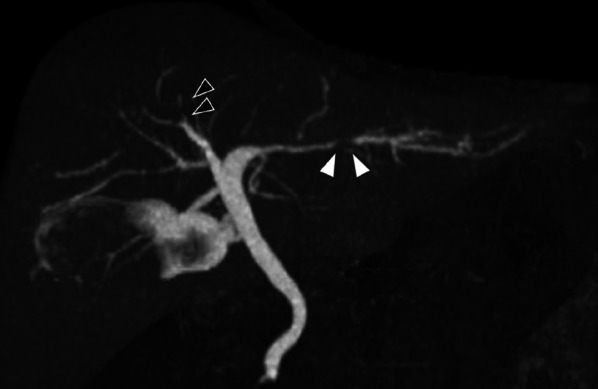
Preoperative DIC-CT findings. Black arrows: interruption and narrowing of the bile duct in the root area of segment B8. White arrows: narrowing of the bile duct in segment B2
